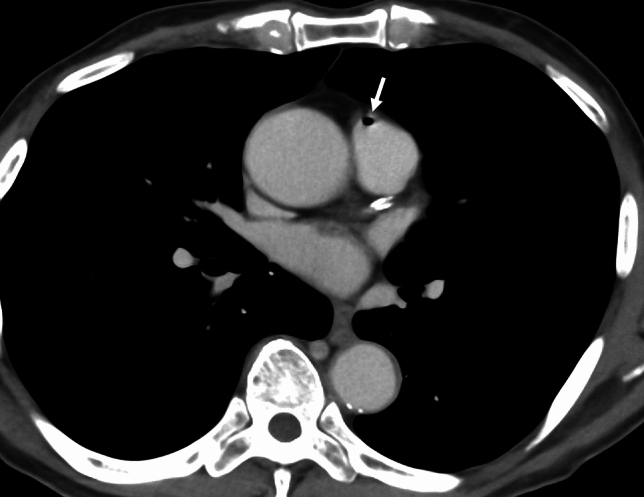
Air embolism after undergoing contrast-enhanced CT. An 80-year-old male underwent an examination by contrast-enhanced CT, which revealed a filling defect with a negative CT value (− 730 HU) in the main pulmonary artery. The amount of air was small and not clinically significant
